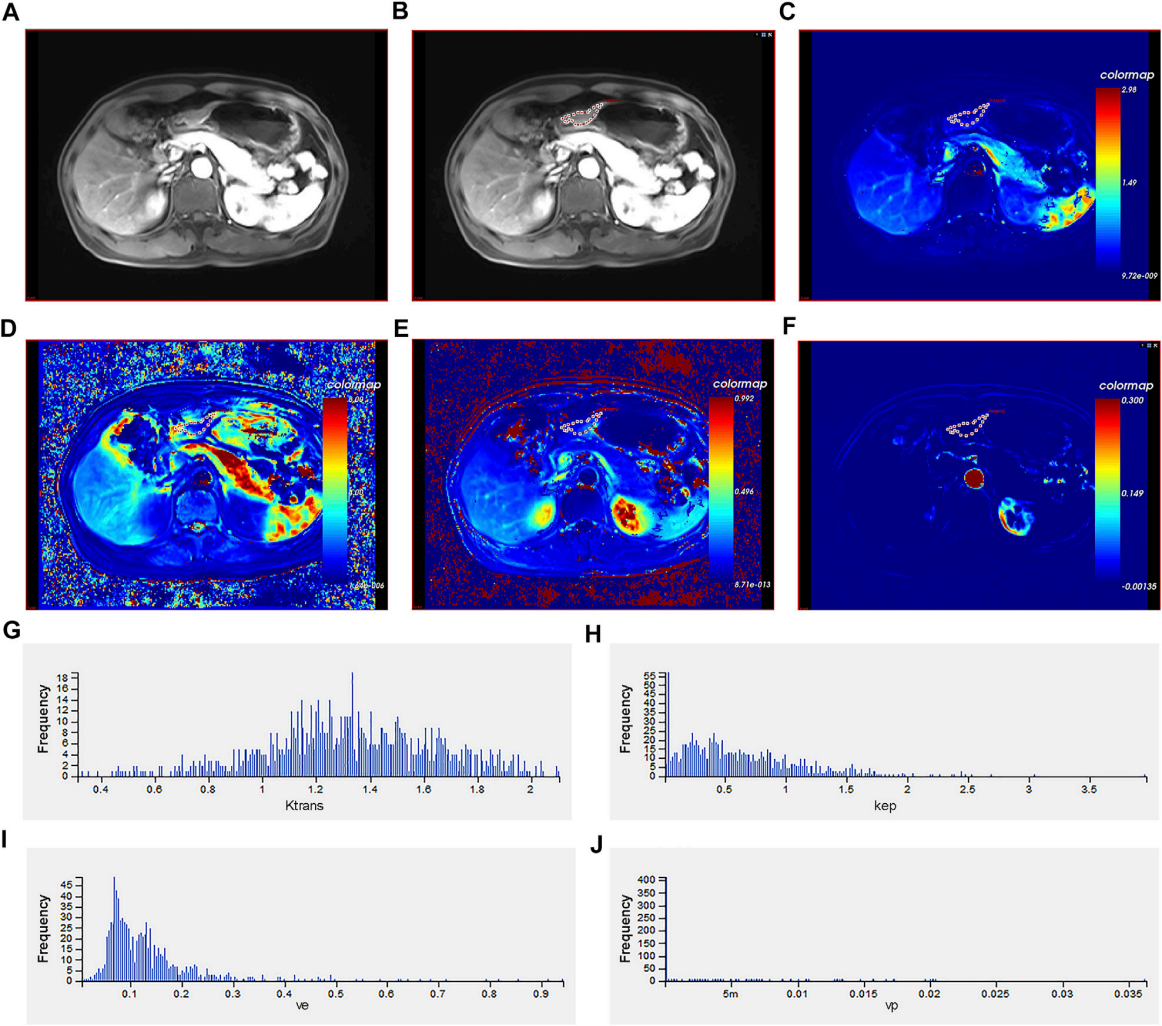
Different imaging modalities and histogram of quantitative perfusion parameters of a 40-year-old male with advanced gastric cancer. (A) Axial T1-weighted image showing a mass with irregular and thickened gastric wall. (B) Manual ROI placement in the axial T1-weighted image. (C) Volume transfer constant (Ktrans) map of ROI. (D) Reverse reflux rate constant (Kep) map of ROI. (E) Extracellular extravascular volume fraction (Ve) map of ROI. (F) Plasma volume fraction (Vp) map of ROI. (G) Histogram of Ktrans values. The histogram analysis parameters (min^−1^) were as follows: skewness = 0.980, kurtosis = 0.303, uniformity = 0.451, energy = 0.009, and entropy = 7.060. (H) Histogram of Kep values. The histogram analysis parameters (min^−1^) were as follows: skewness = 2.271, kurtosis = 9.384, uniformity = 0.226, energy = 0.120, and entropy = 6.050. (I) Histogram of Ve values. The histogram analysis parameters (min^−1^) were as follows: skewness = 2.309, kurtosis = 12.511, uniformity = 0.555, energy = 0.015, and entropy = 6.300. (J) Histogram of Vp values. The histogram analysis parameters (min^−1^) were as follows: skewness = 4.985, kurtosis = 24.264, uniformity = −2.596, energy = 0.761, and entropy = 1.334. ROI: region of interest.
